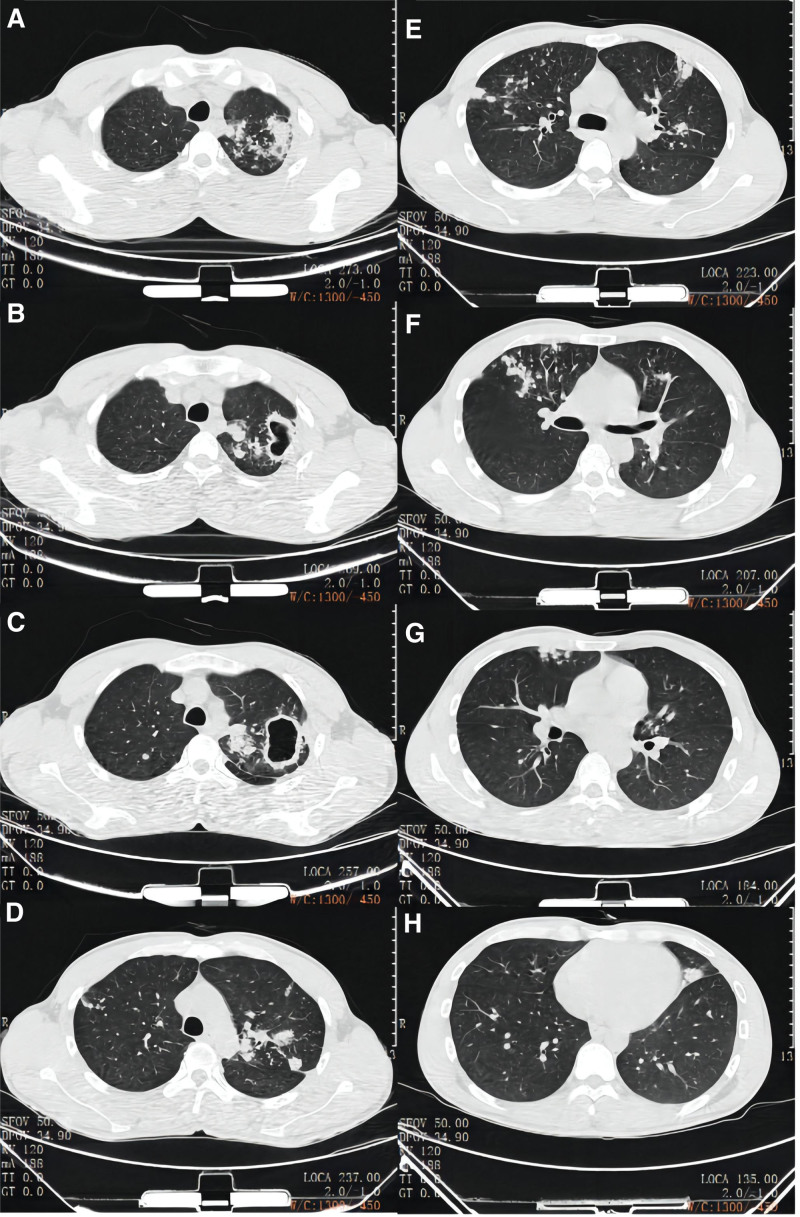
Chest CT of the patient taken on May 5, 2022.
